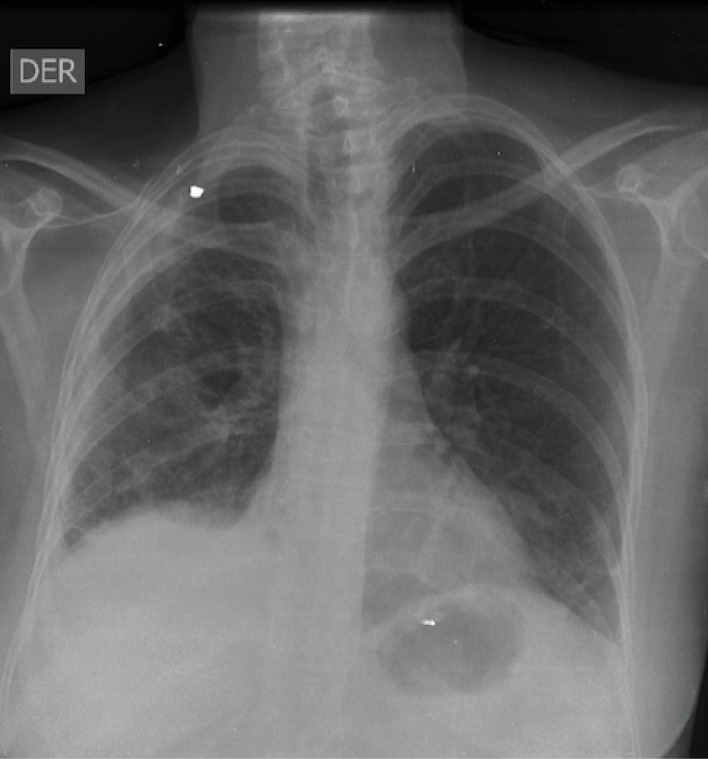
A total of 25 patients (55.5%) had complete resolution of pleural effusions on post-op imaging; complete success, along with symptomatic resolution. Asymptomatic patients having respiratory improvement with residual pleural effusions was 37.7% (17 patients); partial success. Total success rate was 93.3% (42 patients). Of the 25 patients with complete pleural effusion resolution, 36% (9 patients) were male and 64% (16 patients) were female. Follow-up was done on an out-patient basis at one month post-operative with chest x-rays. Because of the advanced oncological stage of the patients, mid- to long- term follow-up was not possible; this holds true to the palliative nature of Pleurodesis.
